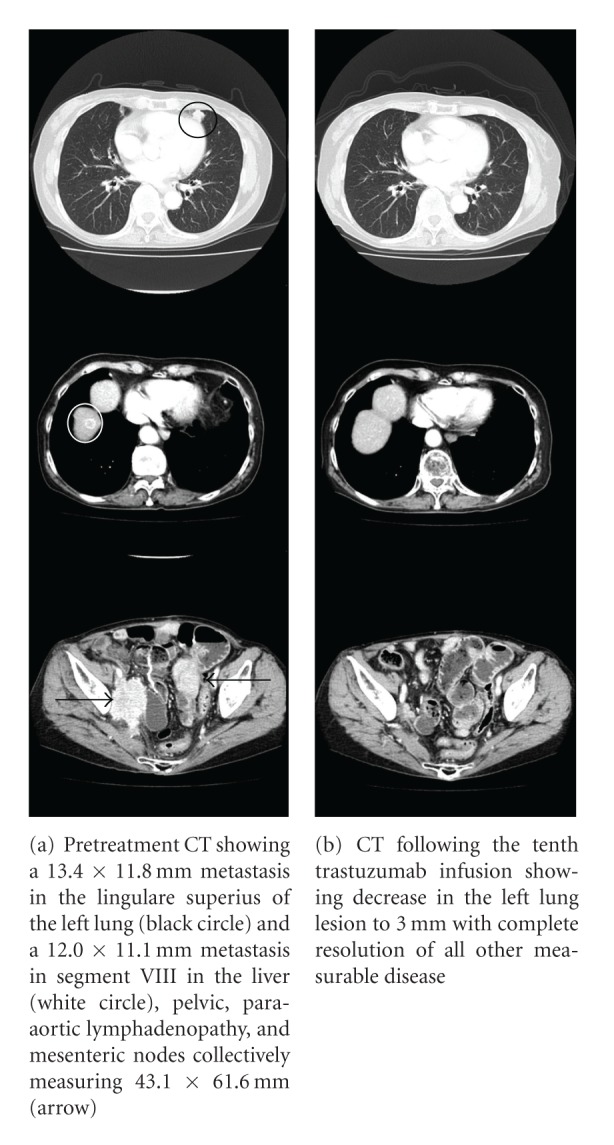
CT image of the metastatic lesion.
